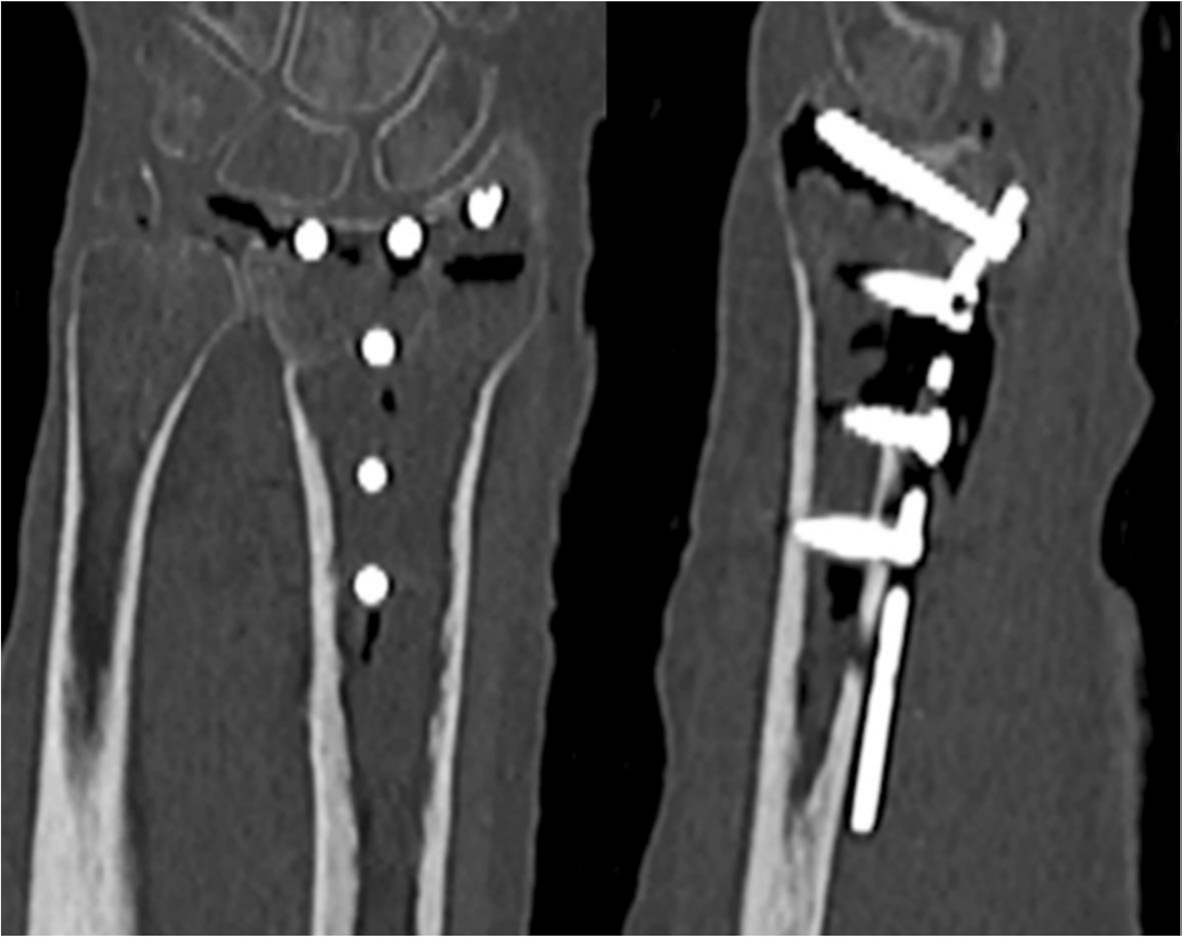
CT images in coronal and sagittal planes
